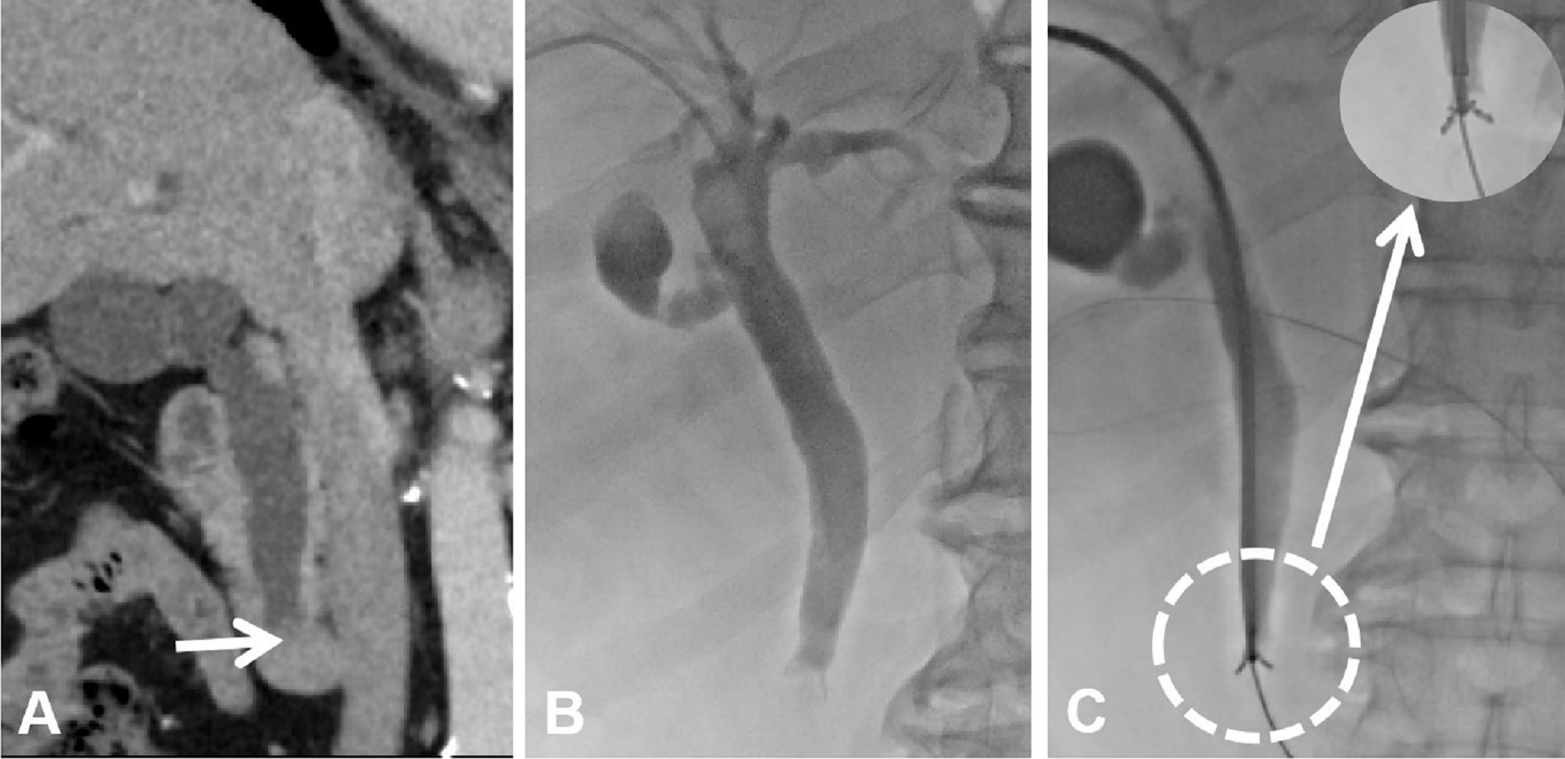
Imaging and biopsy of the biliary obstruction. (A) Coronal enhanced CT scan showing the lower biliary obstruction caused by PC. (B) Percutaneous transhepatic cholangiography was performed to visualize the location and degree of biliary obstruction. (C) Forceps biopsy was performed at the site of the biliary obstruction *via* a 9-F long sheath.
